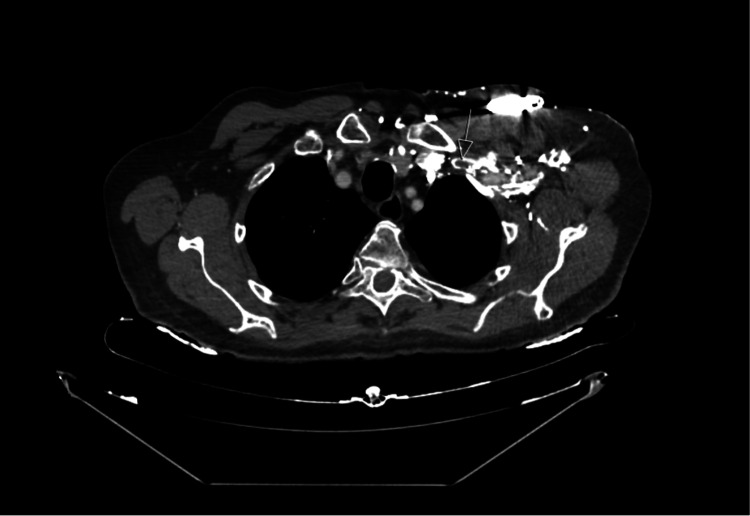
CT TAP confirmed the thrombus within the left subclavian vein (white arrow) CT TAP: CT thorax, abdomen, and pelvis Axial plane
